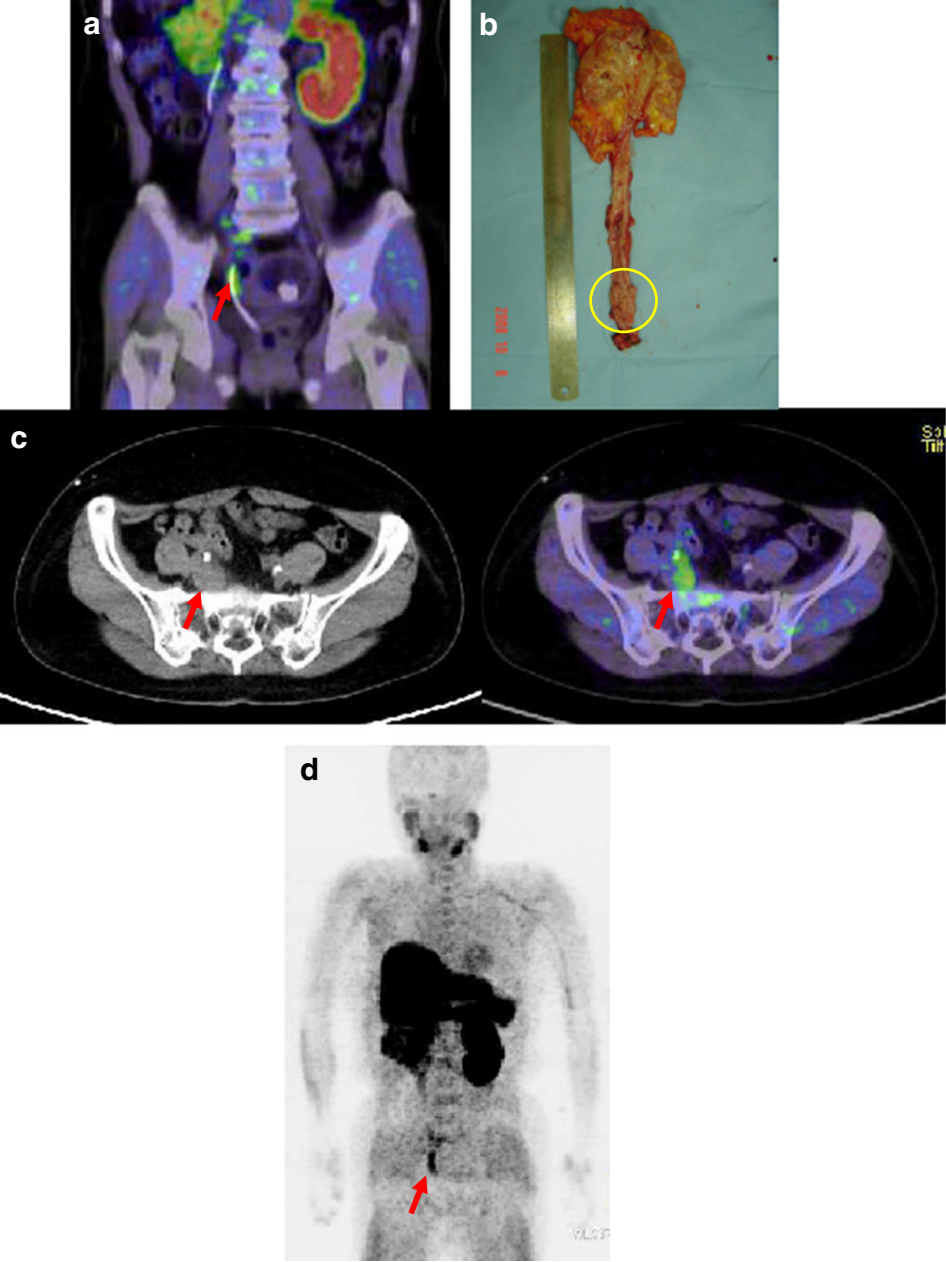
Urothelial carcinoma of the right ureter in patient 11. a Choline PET/CT images of the primary tumour at 10 min after injection (SUVmax 6.09). b Photograph of the primary tumour after resection. *Circle* tumour site. c Choline PET/CT images of the metastatic lesions at the right internal iliac lymph nodes at 10 min after injection (SUVmax 4.92). d Maximum intensity projection image. *Arrows* tumour sites. A catheter is inserted into the right ureter (a, d)
